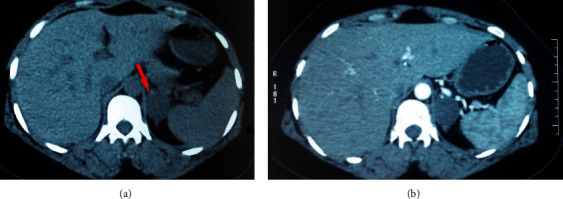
Unenhanced computed tomography scan (a) of the abdomen showing a unilocular left adrenal cyst, without wall's enhancement after contrast injection (b).
